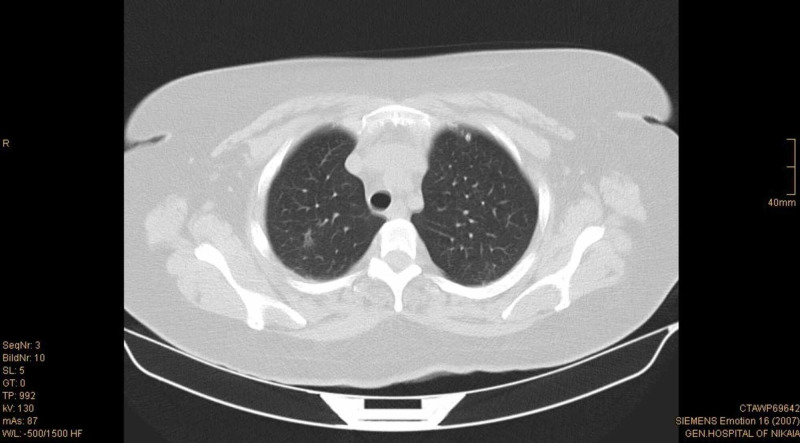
Chest CT scan that shows multiple small round nodules bilaterally.
